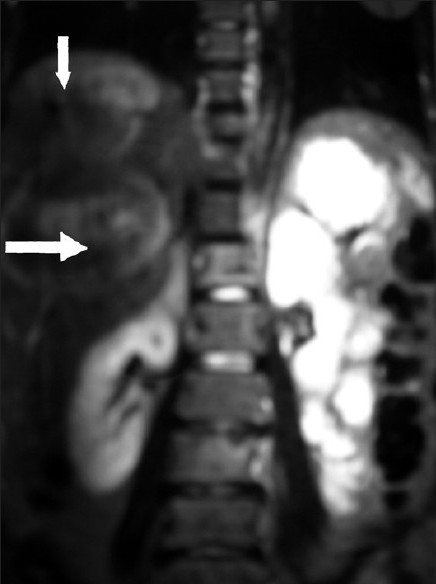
MRI of abdomen showing large space occupying lesions in liver
